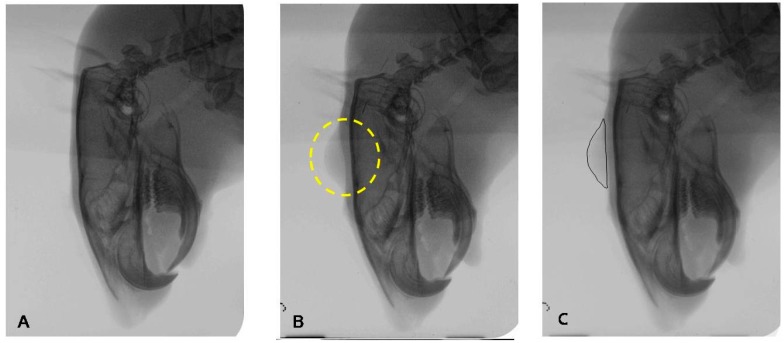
Method for standardizing measurements of cranial subcutaneous injection. (A) Initial lateral cephalometric radiograph before filler injection; (B) Lateral cephalometric radiograph after treatment (circle is the filler injected area); (C) The color of X-ray A was reversed and overlapped to X-ray B (marked circle indicates the augmentation area induced with filler).
